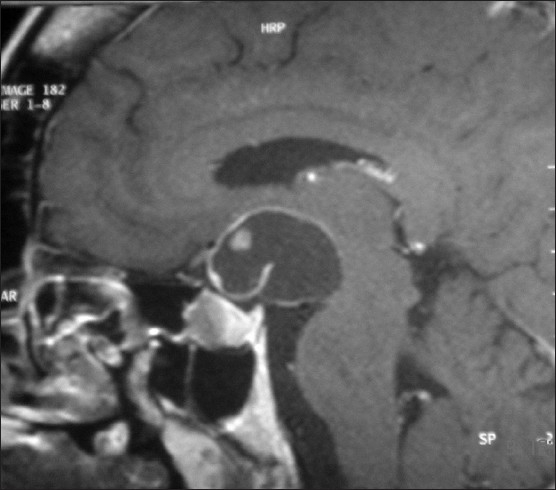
Sagittal MRI brain with enlarged pituitary and suprasellar cyst.
